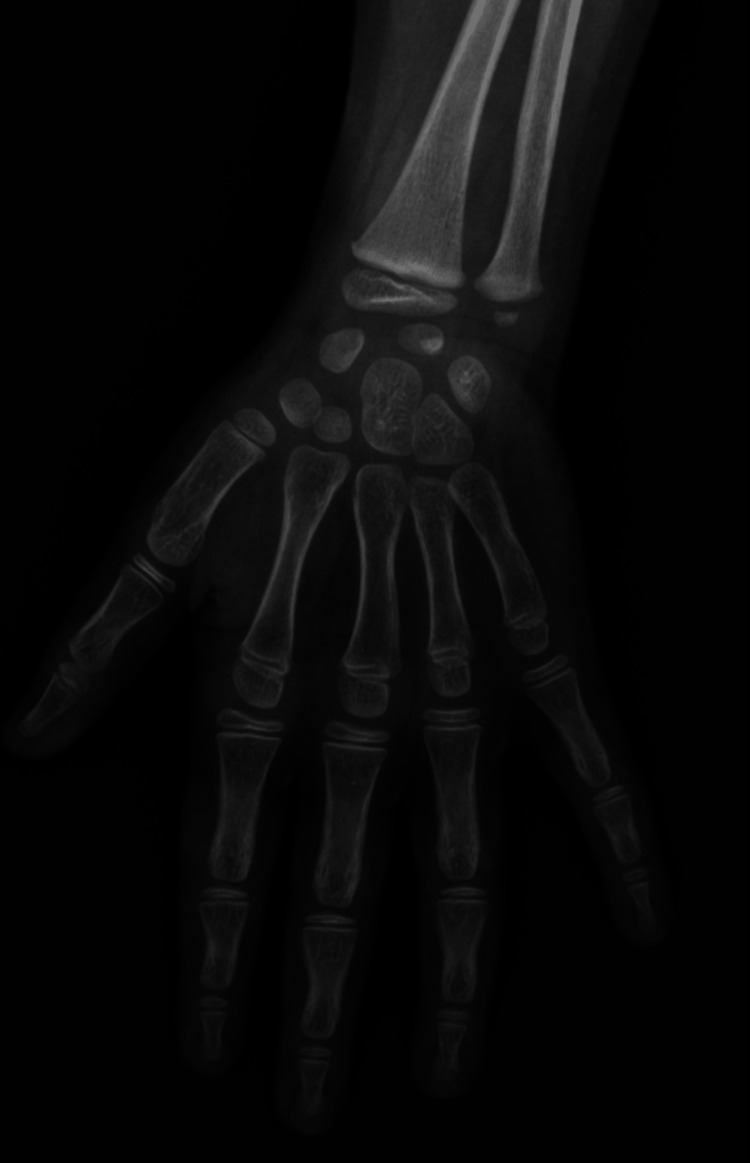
The hand and wrist x-rays of our three-year-old patient demonstrated her bone age at six years.
